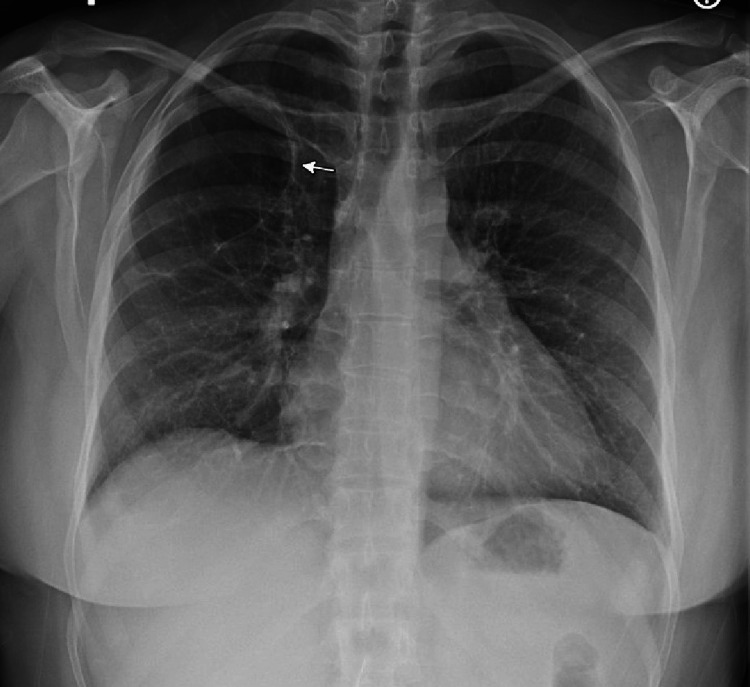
Follow-up Chest Radiograph Two-Weeks Post-ICU Discharge The white arrow indicates a stable large right pneumatocele.
